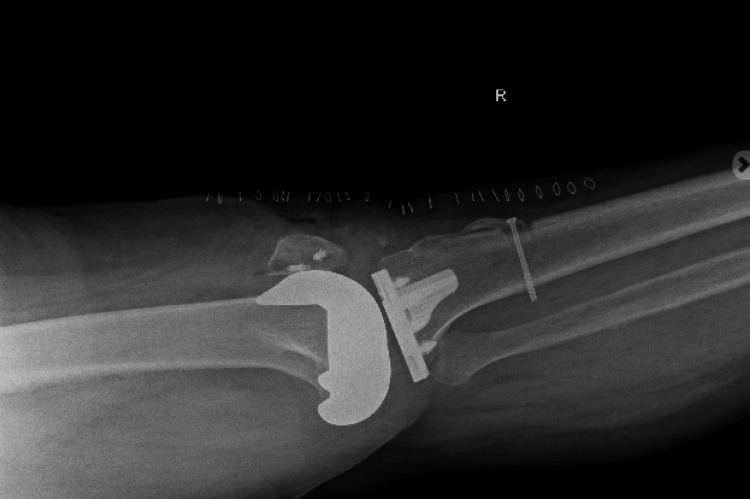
Postoperative lateral radiograph of the right knee Postoperative lateral radiograph of the right knee demonstrating intact total knee arthroplasty components with restored patellar height following Achilles tendon allograft reconstruction of the patellar tendon.
